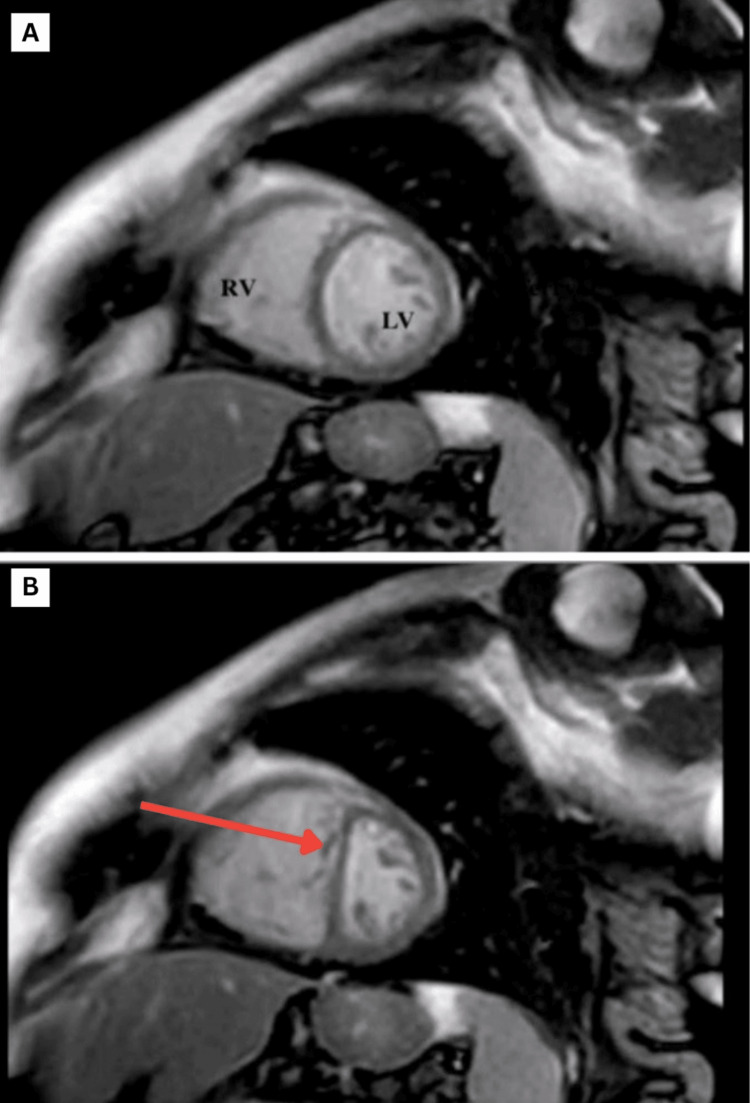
Cardiac magnetic resonance imaging (MRI) Figure A shows a normal interventricular septum in diastole. Figure B shows a leftward shift towards the ventricle known as septal bounce or flattening in inspiration.
